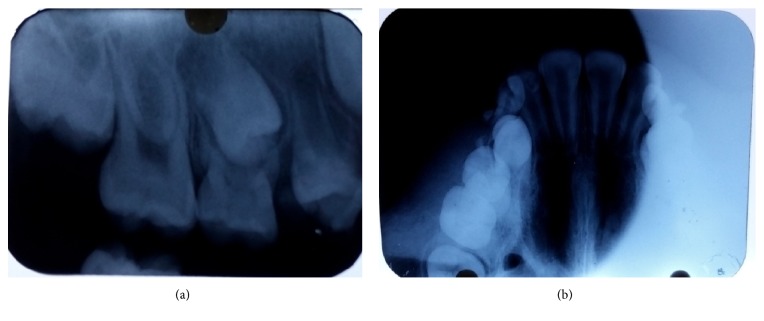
(a) Showing granular trabecular pattern in the IOPA of maxillary right back tooth region. (b) Showing maxillary true occlusal radiograph of same region with granular trabecular pattern, increased buccal cortical expansion, and mild palatal cortical expansion.
